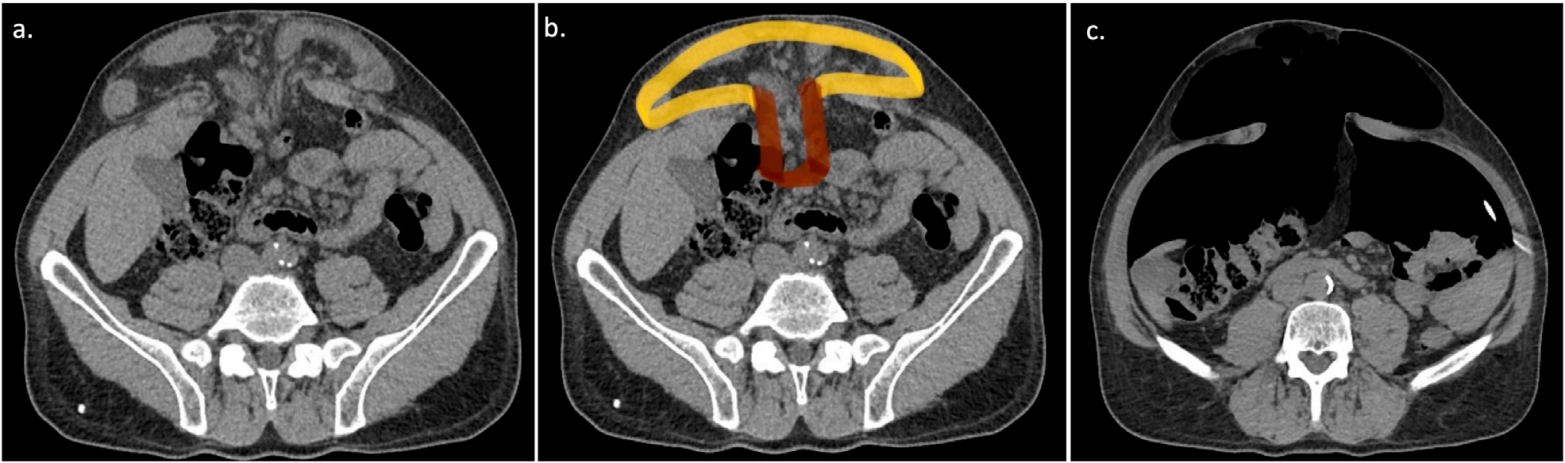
“Mushroom” pattern is characterized by a relatively small hernia defect in hernias with large volume and loss of domain. A CT scan of a complex midline hernia with “mushroom” pattern is shown before (A,B) and after the PPP (C).
